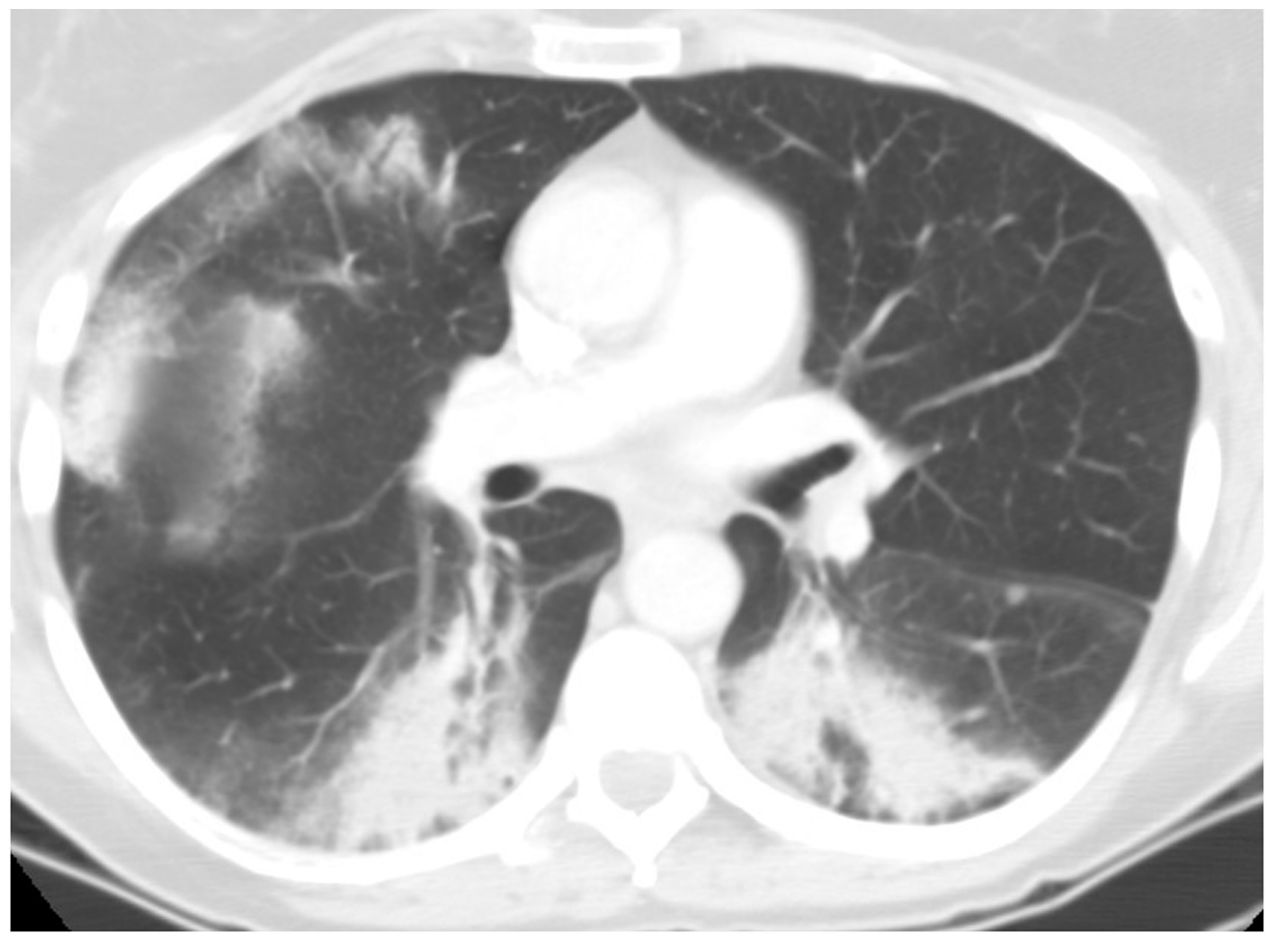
HRCT findings in Loeffler syndrome. Marked fleeting infiltrates associated with bilateral sites of consolidation, ground glass opacities with peri-lobular and peripheral lung distribution.
